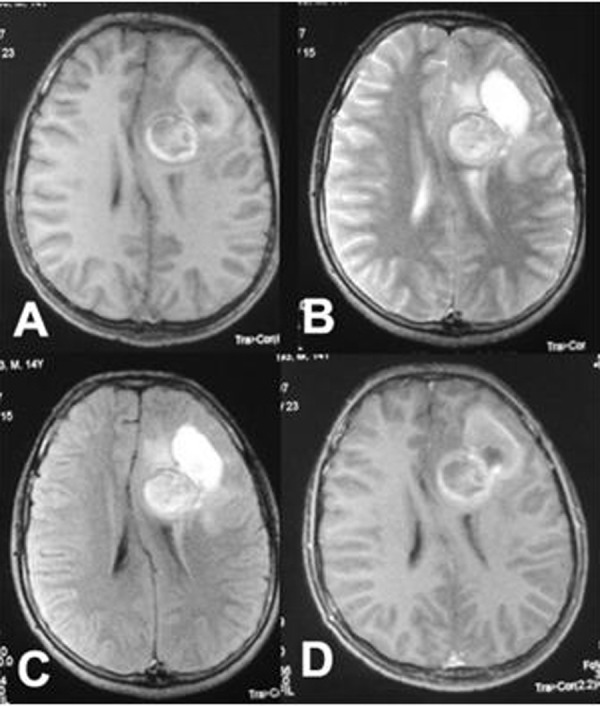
Figure 1: MRI axial section showing well defined lesion in left basal ganglion with evidence of haemorrhage, (A) T1, (B) T2, (C) FLAIR images and (D) minimal enhancement after contrast administration.
